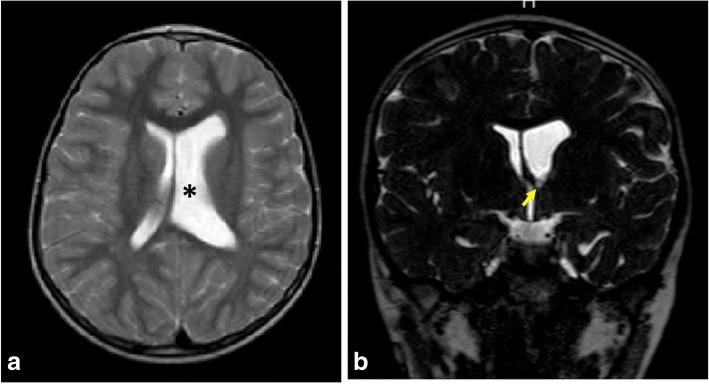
MRI of a 6-year-old boy with foramen of Monro stenosis. a Axial T2-WI showing dilated left lateral ventricle (asterisk). b Coronal 3D-DRIVE demonstrating foramen of Monro stenosis (arrow)
